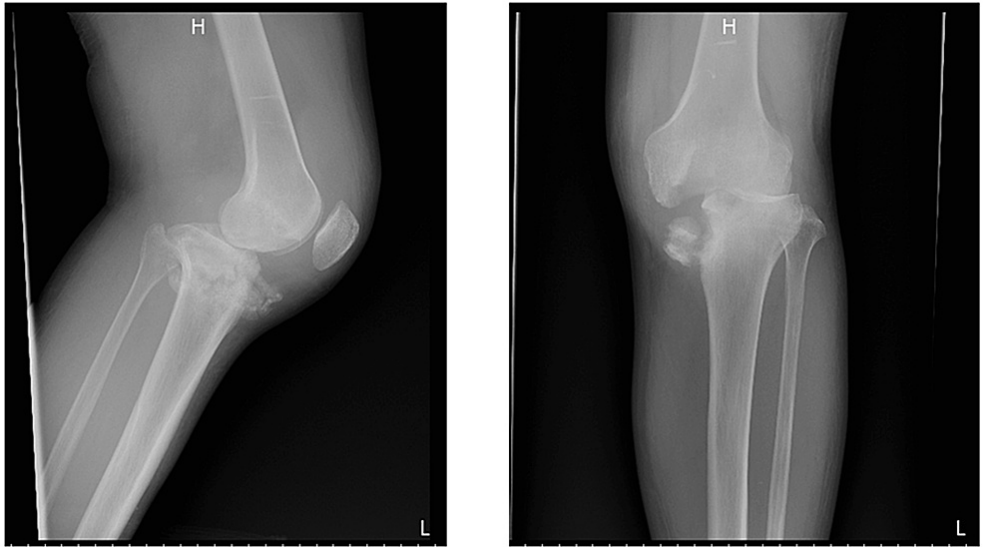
Radiographs of the left knee on admission. Lateral (left) and anteroposterior (right) views show a comminuted fracture of the medial tibial condyle.
